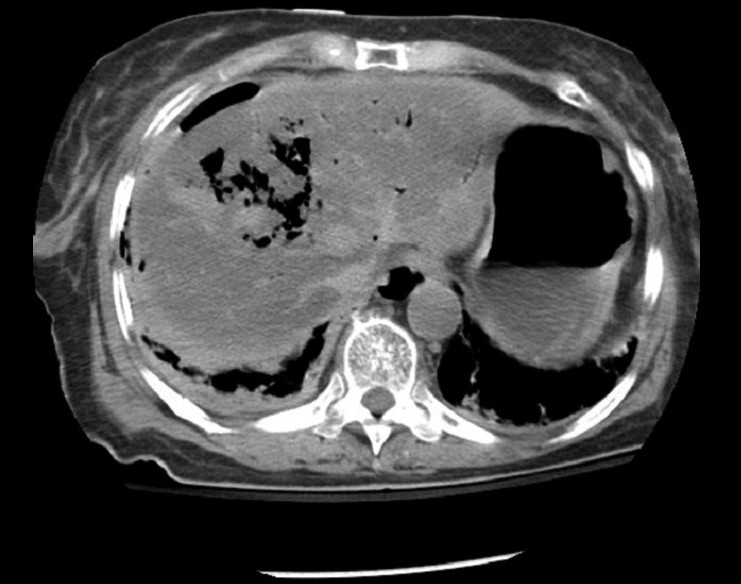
CT scan of the abdomen on patient presentation demonstrating pneumobilia and pneumoperitoneum anterior to the liver
